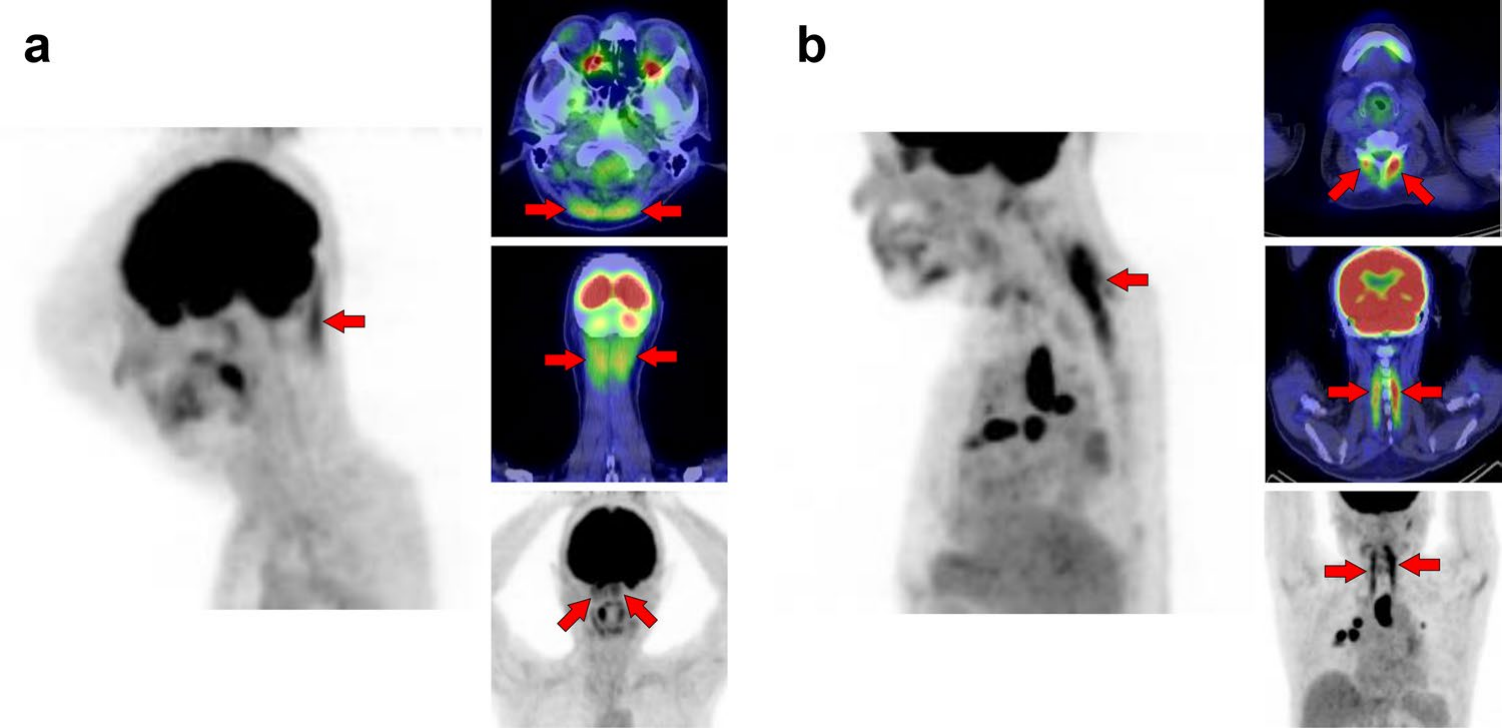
Semispinalis capitis muscle and semispinalis cervicis muscle.* Although strong [^18^F]FDG uptake is occasionally observed, there is usually no specific problem in differentiating from pathological accumulation. Cases of increased uptake in semispinalis capitis muscle (a, arrows) and semispinalis cervicis muscle (b, arrows) are shown
